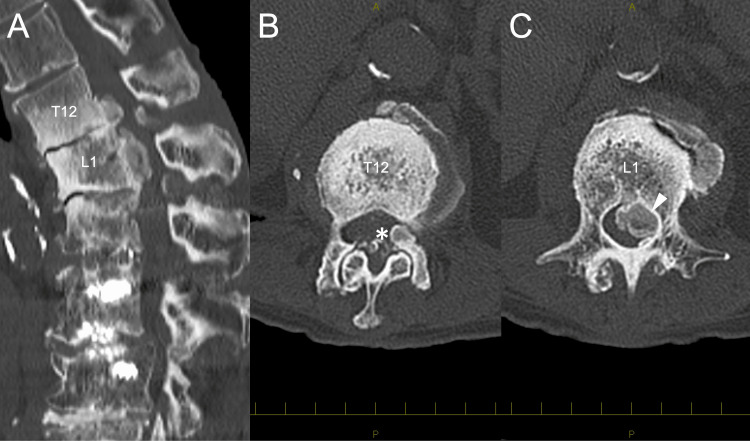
Preoperative CT images (A) Sagittal CT scan reveals the enlarged calcified lesion in ventral epidural space at T12-L1. (B) Axial CT image showsa calcified lesion (indicated by asterisk) at the anterolateral aspect of the left T12-L1 facet joint. (C) Axial CT imageshows the calcified lesion (indicated by white arrowhead) in the ventral epidural space at the L1 level.
